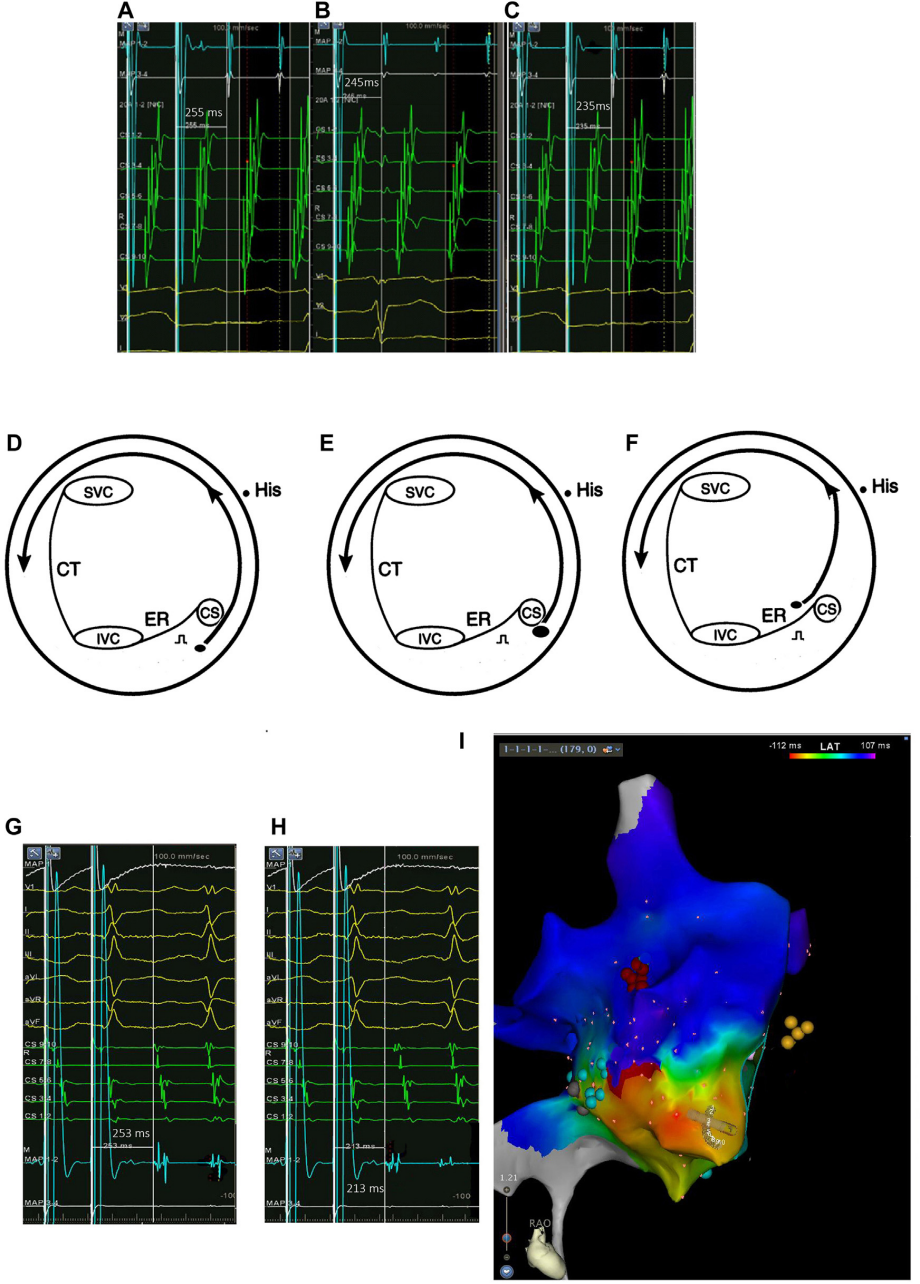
A: Entrainment pacing in the isthmus at a stimulus intensity of 5 mA with a postpacing interval (PPI) of 255 ms. The tachycardia cycle length (TCL) was 255 ms, and pacing rate was 245 ms. B: Entrainment pacing in the isthmus at a stimulus intensity of 8 mA with a PPI of 246 ms. C: Entrainment pacing in the isthmus at a stimulus intensity of 10 mA with a PPI of 235 ms. D: Pacing at lower intensity. The virtual electrode *(oval)* is next to the pacing site. E: Pacing at higher intensity. The virtual electrode is farther away. F: If pacing near the scar or the area of conduction block (eg, eustachian ridge [ER]), the pacing virtual electrode may capture one or the other side of the barrier side. The circuit may be changed and the PPI changed significantly. G: Pacing at lower intensity. PPI was 253 ms, TCL was 220 ms, and pacing rate was 210 ms. H: Pacing at higher intensity. PPI was 213 ms. Note the double potentials recorded at the pacing electrode. I: Three-dimensional mapping showing typical atrial flutter. *Yellow tags* represent the His bundle; *red tags* represent ablation sites; *black tags* represent scars; and *blue tags* represent double potentials. CS = coronary sinus; CT = crista terminalis; IVC = inferior vena cava; SVC = superior vena cava.
